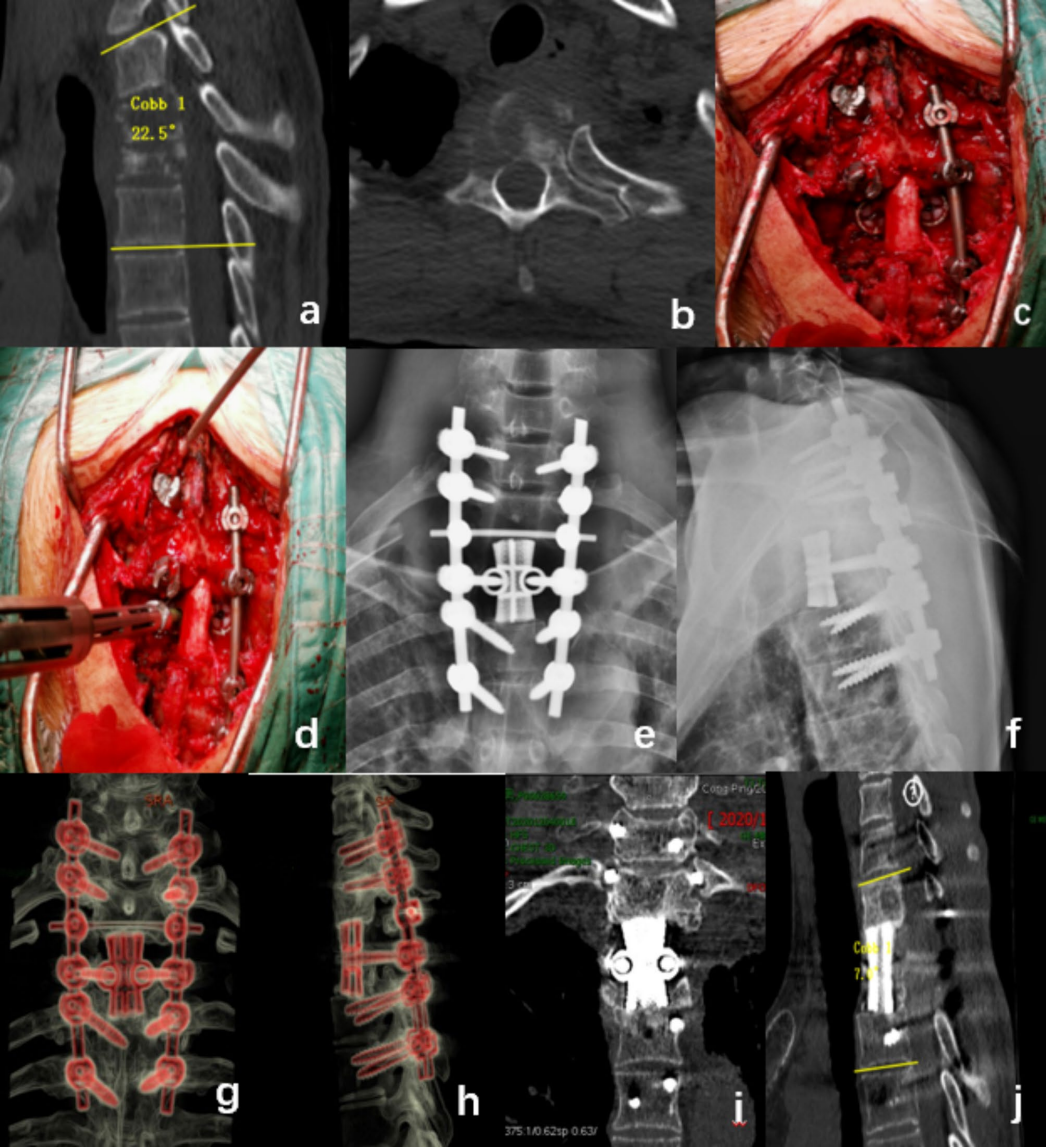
a 38-year-old male patient with pulmonary cancer exhibiting T2 and T3 metastases. Preoperative CT scans of the patient revealed a kyphotic deformity of the thoracic spine (a.b). During surgery, a customized artificial vertebral bodies is used to replace the excised vertebrae (c.d). The postoperative X-ray shows that the artificial vertebral body is well positioned (e.f). 28 months post-surgery, the X-ray and CT images show that the artificial vertebral body is well-positioned without any subsidence or loosening
